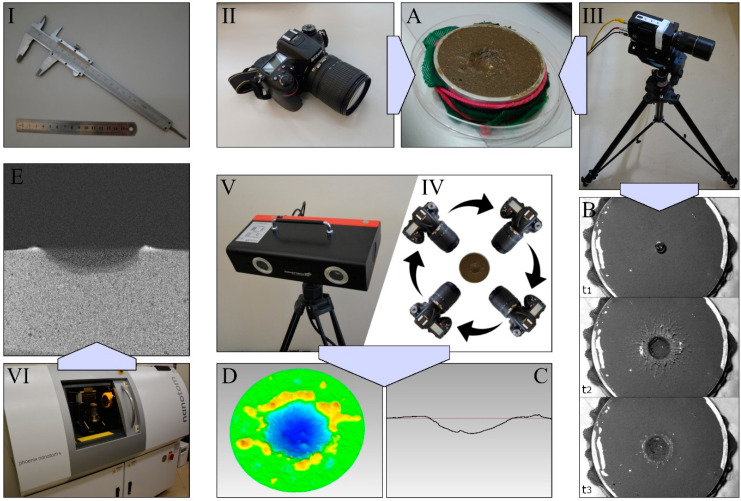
Measurement equipment and methods of analysing data acquired with its help. Measurement equipment: (I)–basic manual measurement methods using, among others, a ruler or calliper; (II)–camera; (III)–high-speed camera; (IV)–devices making it possible to record images for photogrammetry (e.g., cameras); (V)–surface scanners and profilometers; (VI)–computed tomograph. Data analysis methods: (A)–photo analysis; (B)–record analysis; (C)–profile analysis; (D)–3D surface model analysis; (E)–tomographic image analysis.
